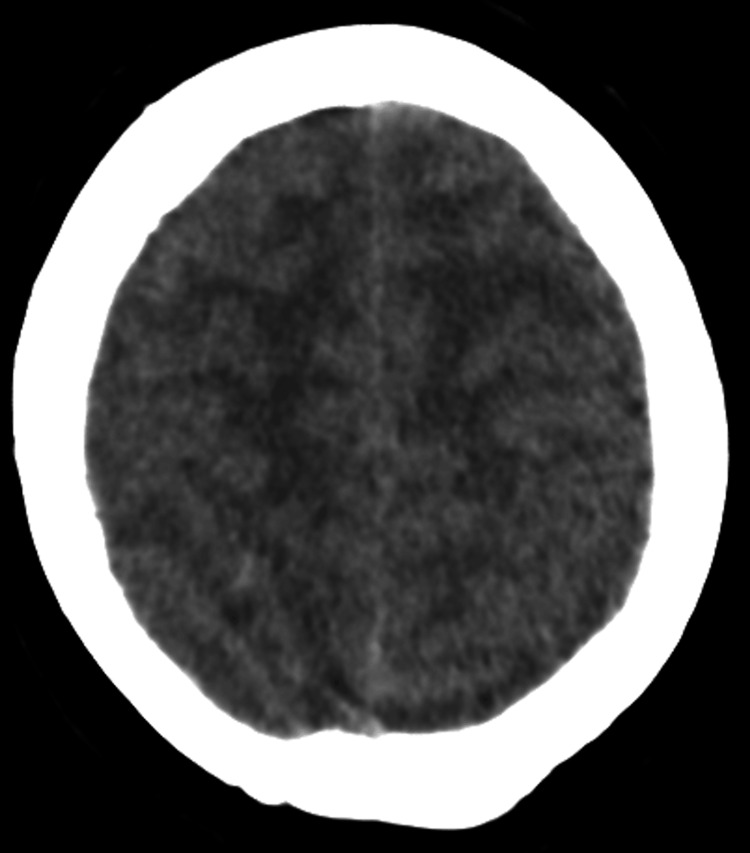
Non-contrast CT Head (axial view) demonstrating generalised sulcal effacement and obliteration of the ambient cisterns.
